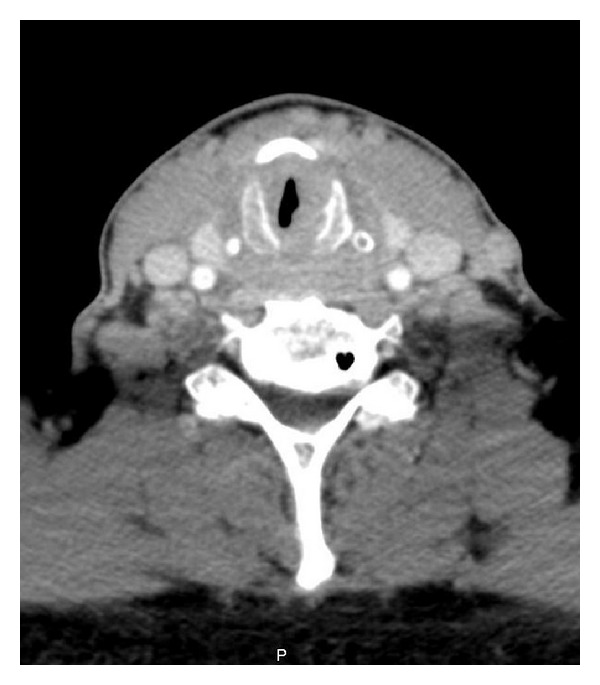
CT scan of the neck. Axial view showing subglottic edema and granuloma tissue on the left posterior commissure.
